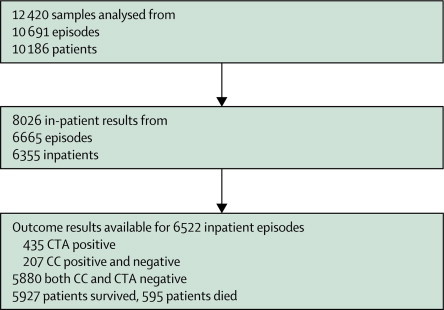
Patient and sample selection CC=cytotoxigenic culture. CTA=cytotoxin assay.
